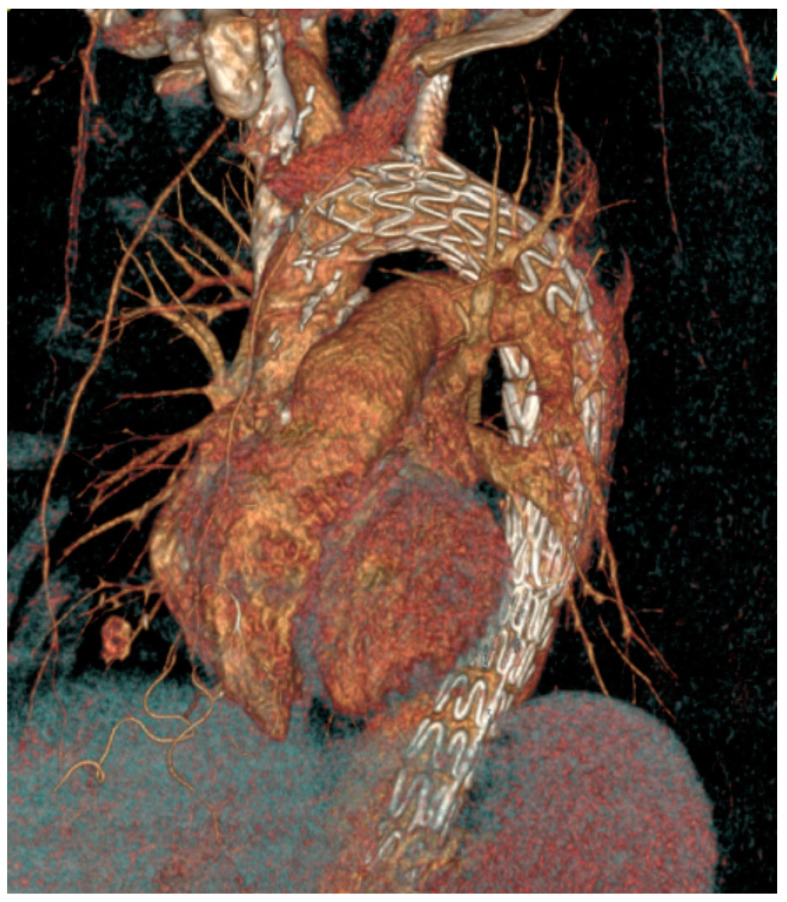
Postoperative computed tomography angiography (CTA) 3D image after the in situ needle fenestration thoracic endovascular aortic repair (TEVAR) procedure.
